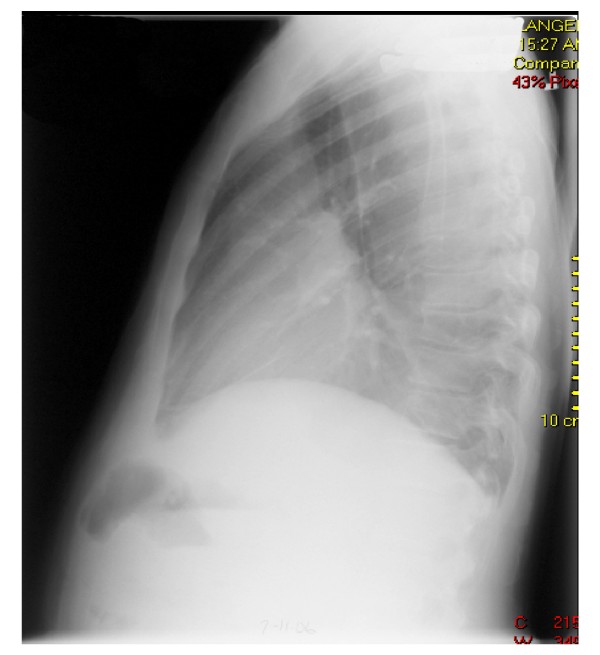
Chest X-ray (lateral view): Re-accumulation of massive left sided pleural effusion.
